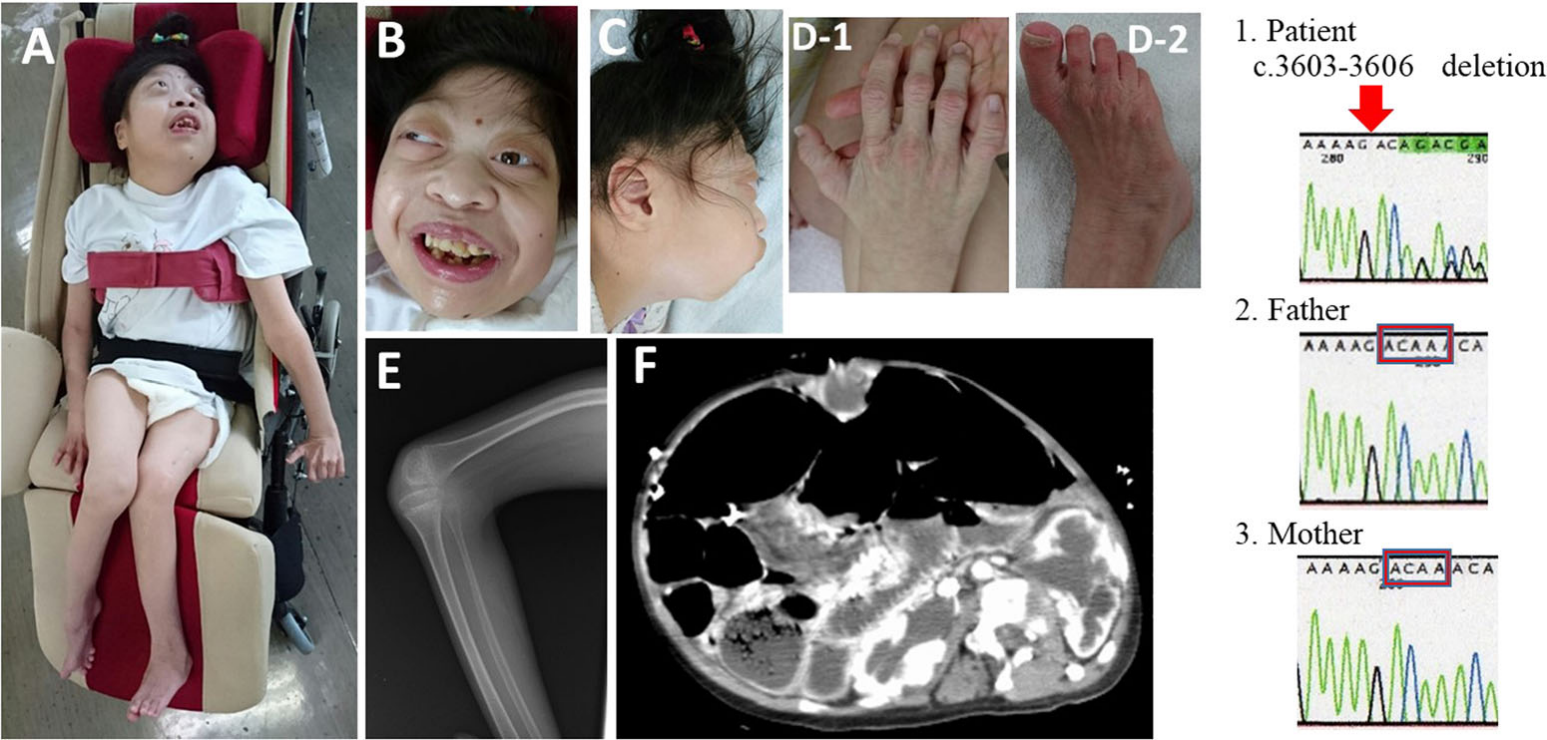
Current characteristic features of the patient and genetic analysis results. a Entire body of the patient. Developmental disturbance and right clubfoot are noted. b Coarse face with proptosis, right strabismus, and prominent broad nose. c Microcephaly and retromicrognathia. d Prominent middle and distal interphalangeal joints, wrinkled extremities, and broad thumb fingernails. e X-ray at 14 years of age reveals agenesis of patellae. f Contrast-enhanced computed tomography of the ileus at 15 years of age reveals pyelectasis and thin renal parenchyma. 1, 2, 3 Sanger sequencing reveals a c.3603-3606 deletion, a de novo, heterogeneous mutation in this patient
